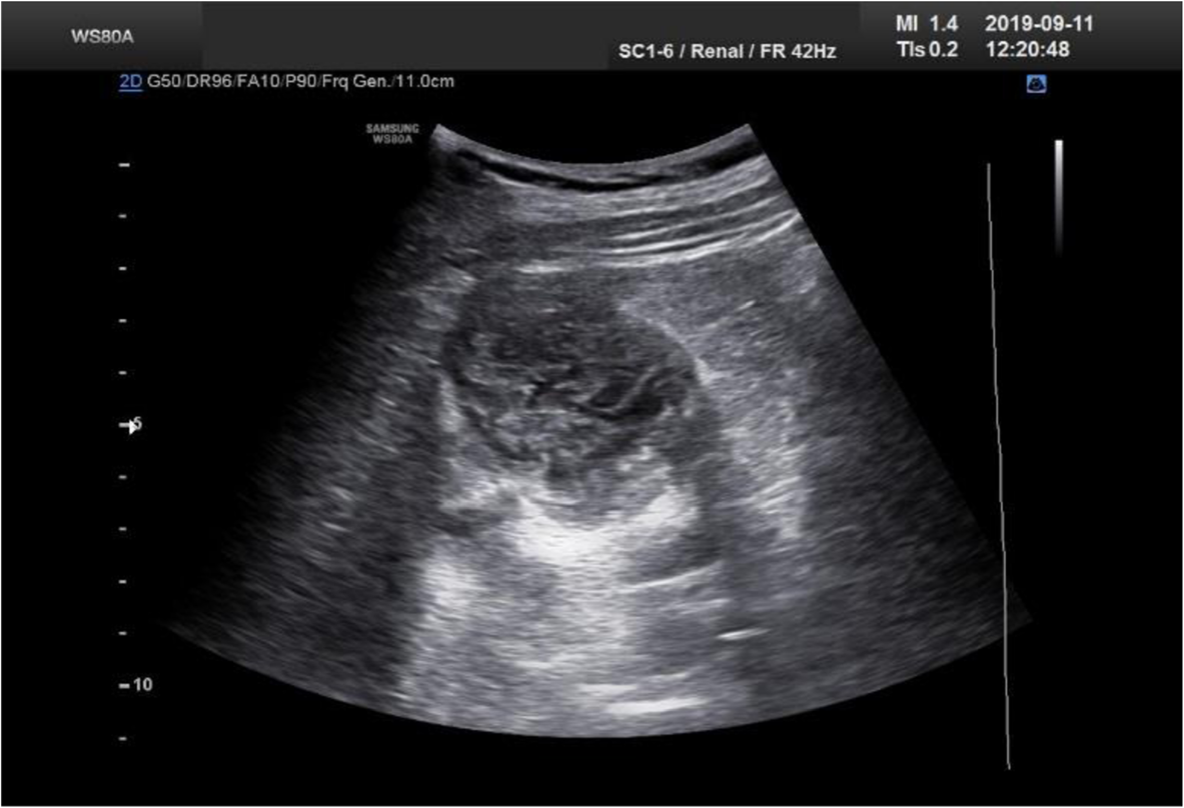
Ultrasonographic evaluation 3 years after discontinuation of medications showing disappearance of daughter cysts
